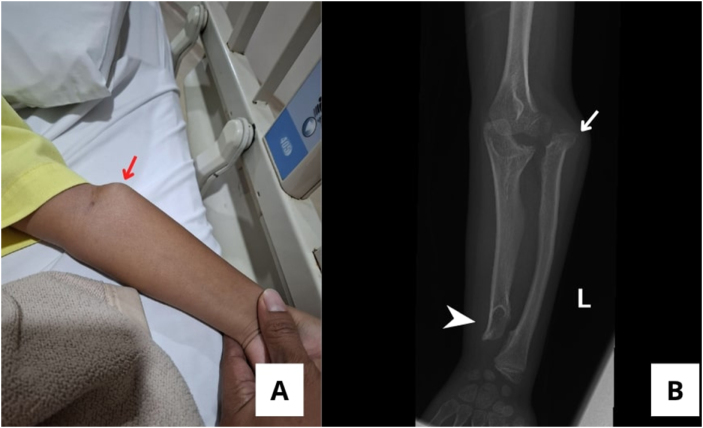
(A) A pre-operative clinical presentation showing a small, firm, and non-tender nodule in the left elbow (red arrow). (B) Plain radiograph AP view of the left forearm showing anterolateral dislocation of the radial head (white arrow) and a shortening of the ulnar (white head arrow).
